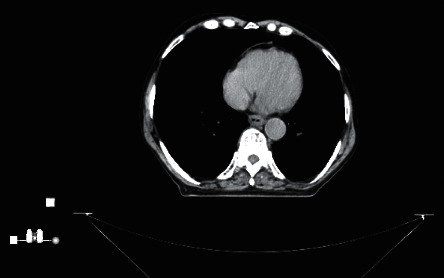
Pretreatment computed tomography showing circumferential esophageal wall thickening located at the distal third of the esophagus.
